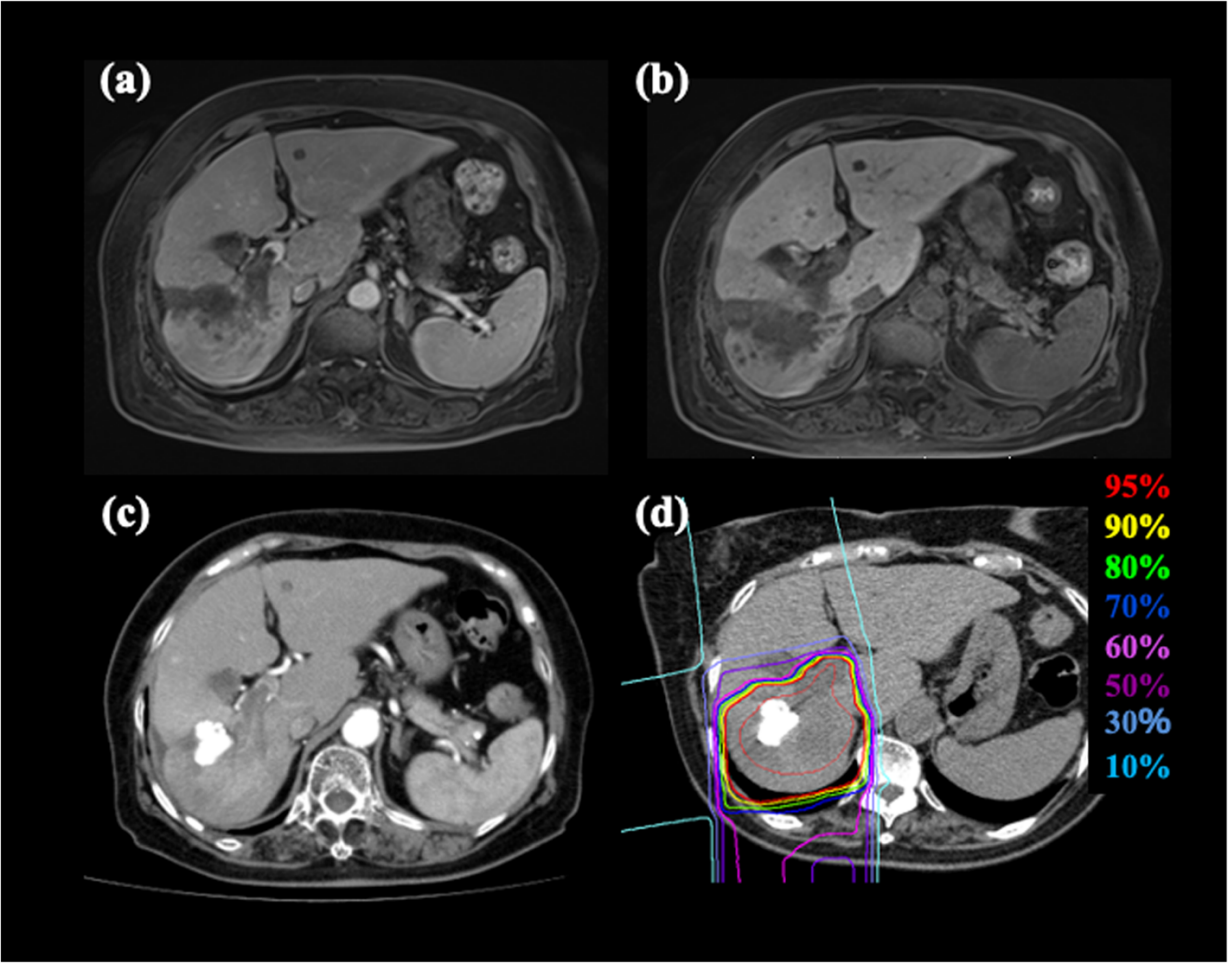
HCC in an 83-year-old female patient treated with C-ion RT. a MRI (early phase) before treatment. b MRI (late phase) before treatment. c CT (early phase) before treatment. d Dose distribution on axial CT images. Highlighted are: 95% (red), 90% (yellow), 80% (green), 70% (blue), 60% (pink), 50% (purple), 30% (light purple), and 10% (light blue) isodose curves (100% = 60 Gy [RBE]). The area within the red outline is GTV. CT, computed tomography; GTV, gross tumor volume; RBE, relative biological effectiveness
